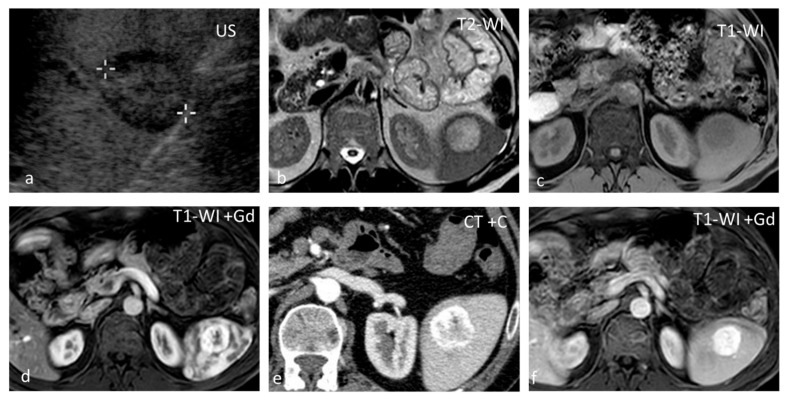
Hemangioma. A well-defined isoechoic splenic lesion with a hypoechoic rim on US (a). The lesion appears hyperintense on T2-WI with a thin hypointense rim (b) and hypointense on T1-WI (c), demonstrating strong peripheral enhancement in the early phases post intravenous contrast administration both at MRI (d) and CT (e), and homogeneous delayed enhancement (f).
